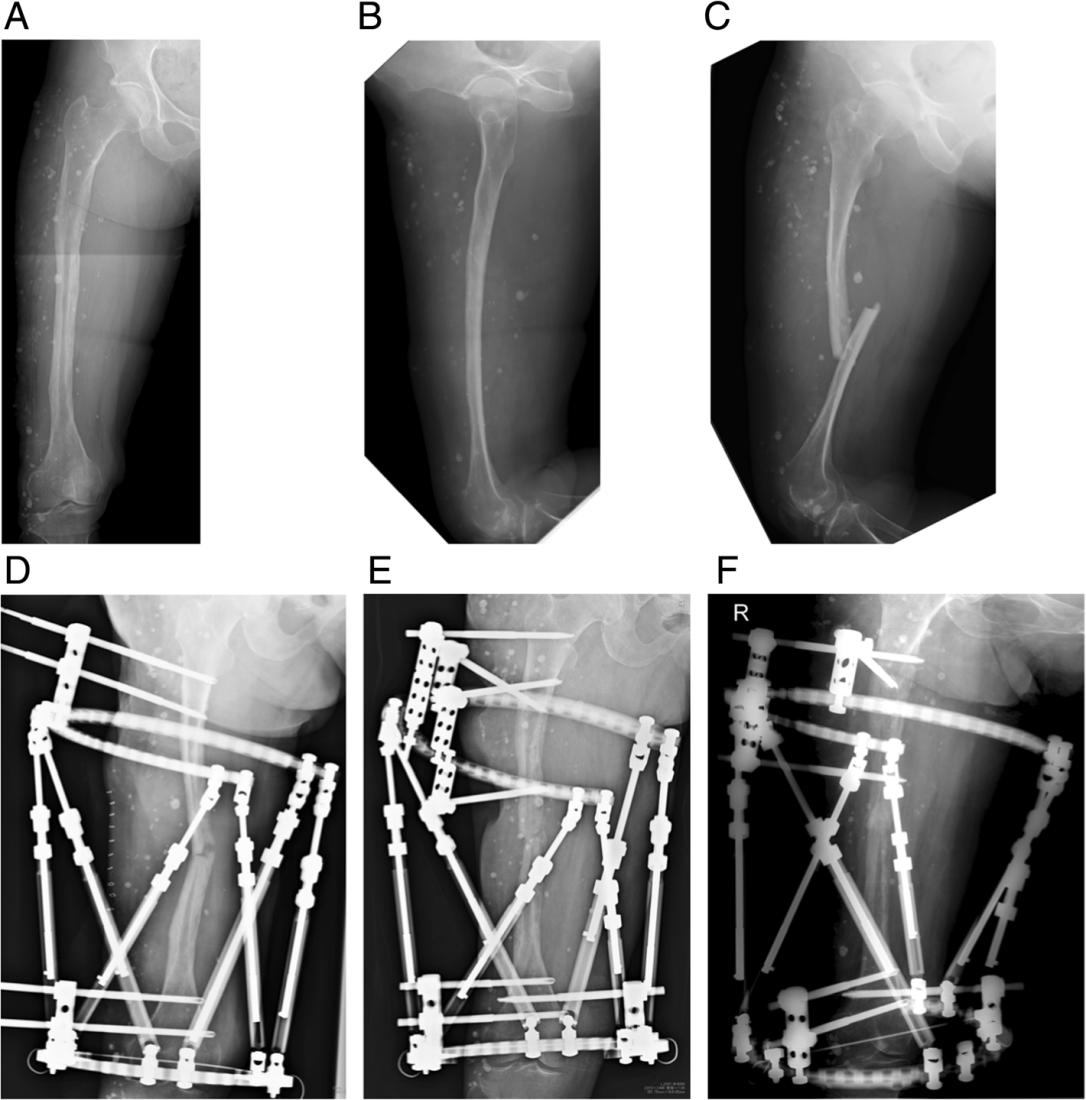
Pre- and postoperative radiographs of the right thigh. Anterolateral (a) and lateral (b) radiographs taken at the first visit at 63 years of age reveal soft tissue swelling containing numerous phleboliths and bony erosion in the femoral shaft. c Radiograph taken on admission at 68 years of age reveals displaced pathologic right femoral shaft fracture. d Radiograph taken immediately after the first surgery reveals partial reduction of the fracture using a Taylor Spatial Frame. e Radiograph taken immediately after the second surgery reveals replacement of the proximal half-pins and complete reduction of the fracture. f Radiograph taken 5 months after the second surgery reveals bony union. One of the proximal half-pins was removed 1 month after the second surgery due to loosening
